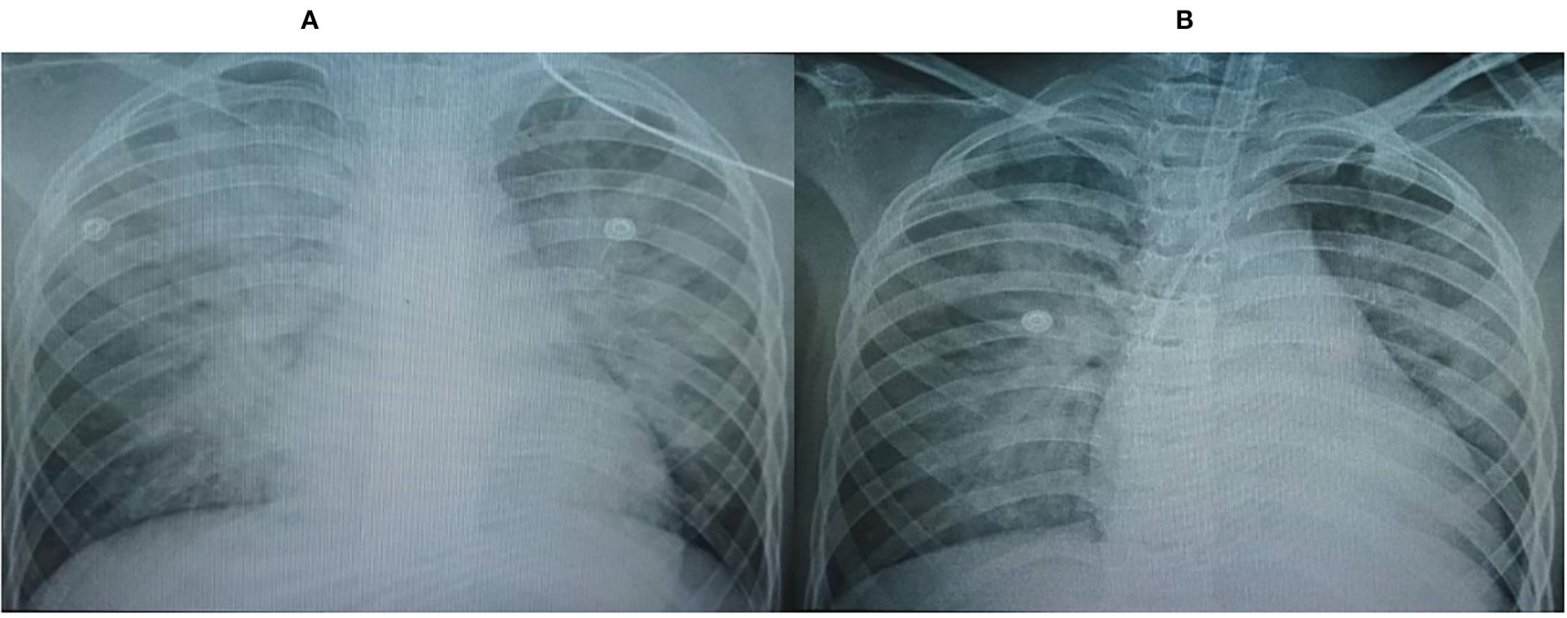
Chest x-rays (CXRs) revealed pulmonary opacification of the bilateral perihilar region and cardiomegaly with a mildly increased cardiothoracic ratio. (A) On admission, (B) Post-24 h hemofiltration.
